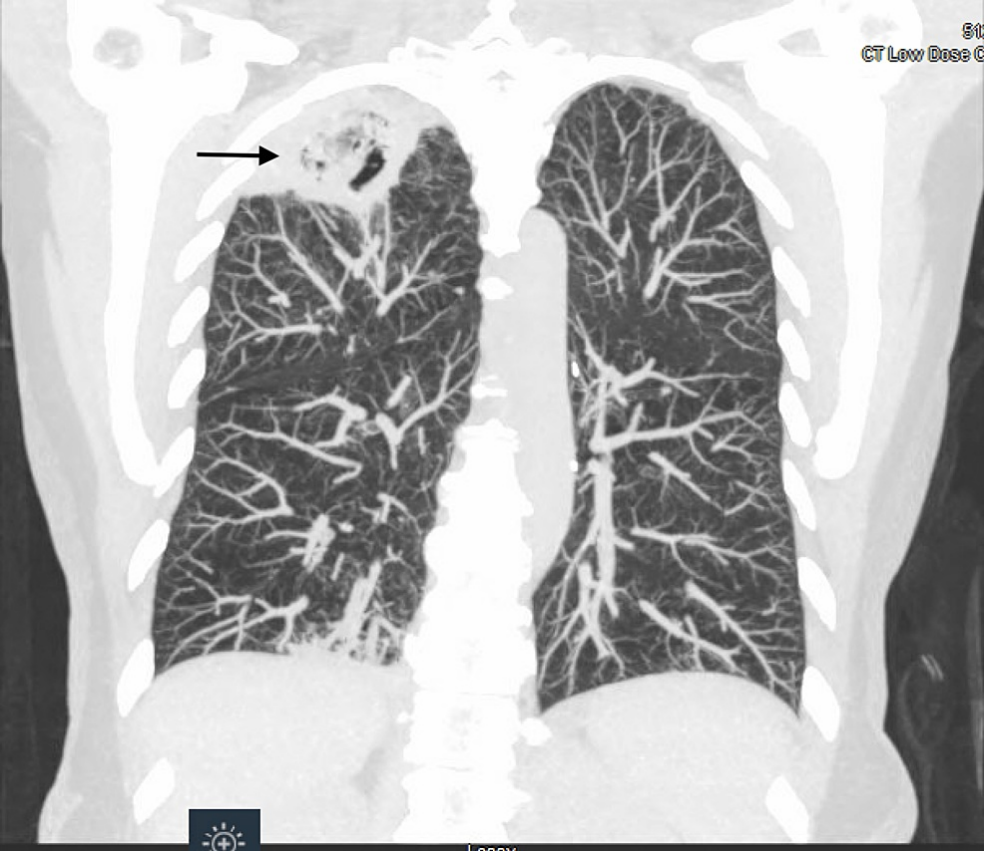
Coronal view of the Low dose CT chest showing a right apical cavitary lesion
